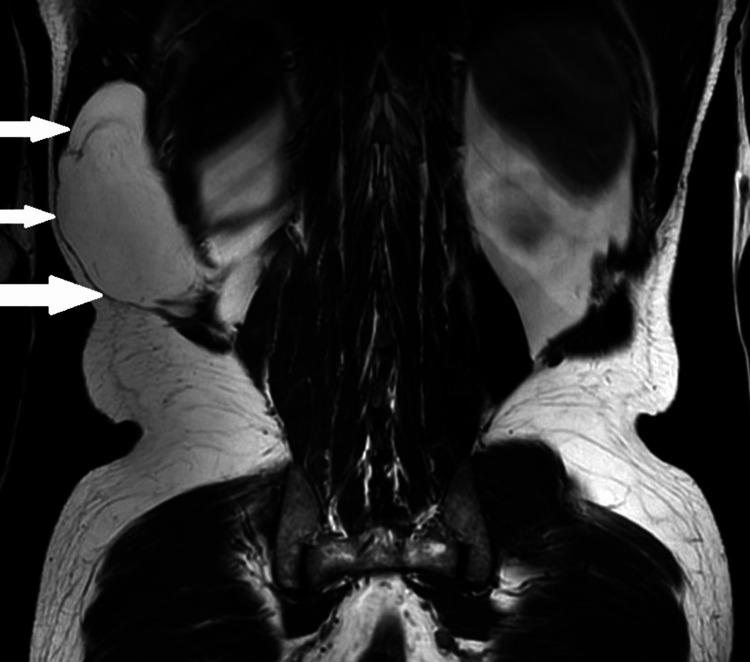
MRI showing intramuscular lipoma MRI: Magnetic resonance imaging MRI shows an encapsulated mass within the right latissimus dorsi muscle, with white arrows pointing toward the intramuscular lipoma
